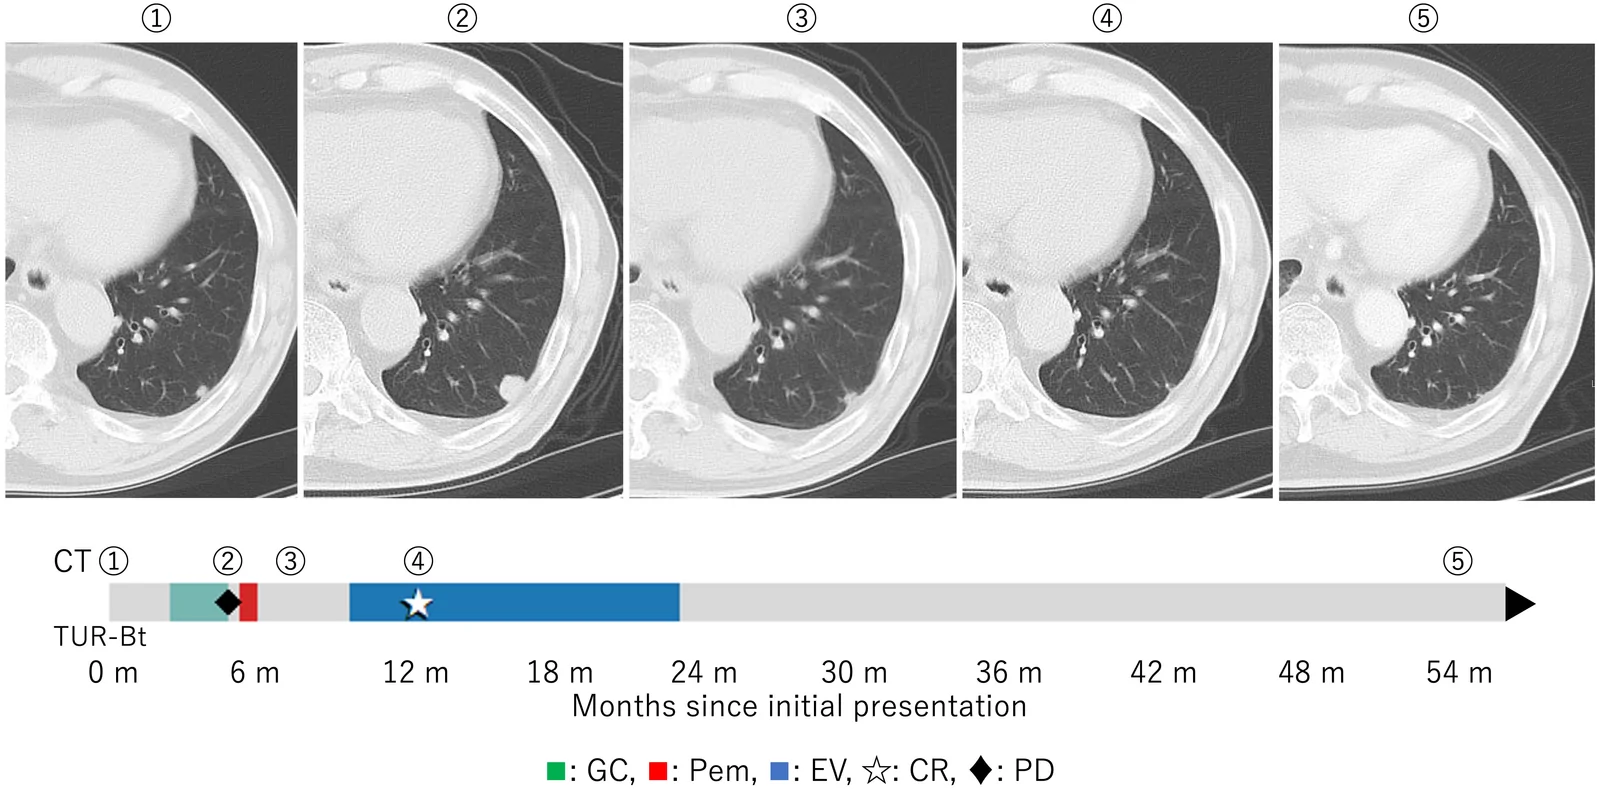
Clinical course and radiographic response. Timeline of treatment and computed tomography (CT) findings for pulmonary metastases from initial presentation to ongoing complete response (CR). Baseline CT showed a bladder tumor with lung metastases (①). Following transurethral resection of bladder tumor (TUR-Bt), pathology confirmed invasive high-grade urothelial carcinoma (≥pT2). The patient received gemcitabine plus cisplatin (GC) for two cycles and was assessed as progressive disease (PD) due to enlargement of lung metastases (②). Pembrolizumab (Pem) was administered as second-line therapy but discontinued after one dose because of destructive thyroiditis. Post-Pem CT demonstrated shrinkage of lung metastases (③). Enfortumab vedotin (EV) was subsequently initiated, and CR was achieved after three cycles (④); EV was continued for a total of 10 cycles with maintained CR. EV was discontinued due to adverse events, and the patient has since been followed under surveillance only. At >4.0 years from Pem initiation, CR remains ongoing (⑤). Symbols: squares indicate systemic therapies (GC, pembrolizumab, EV), diamond indicates PD, and star indicates CR.
